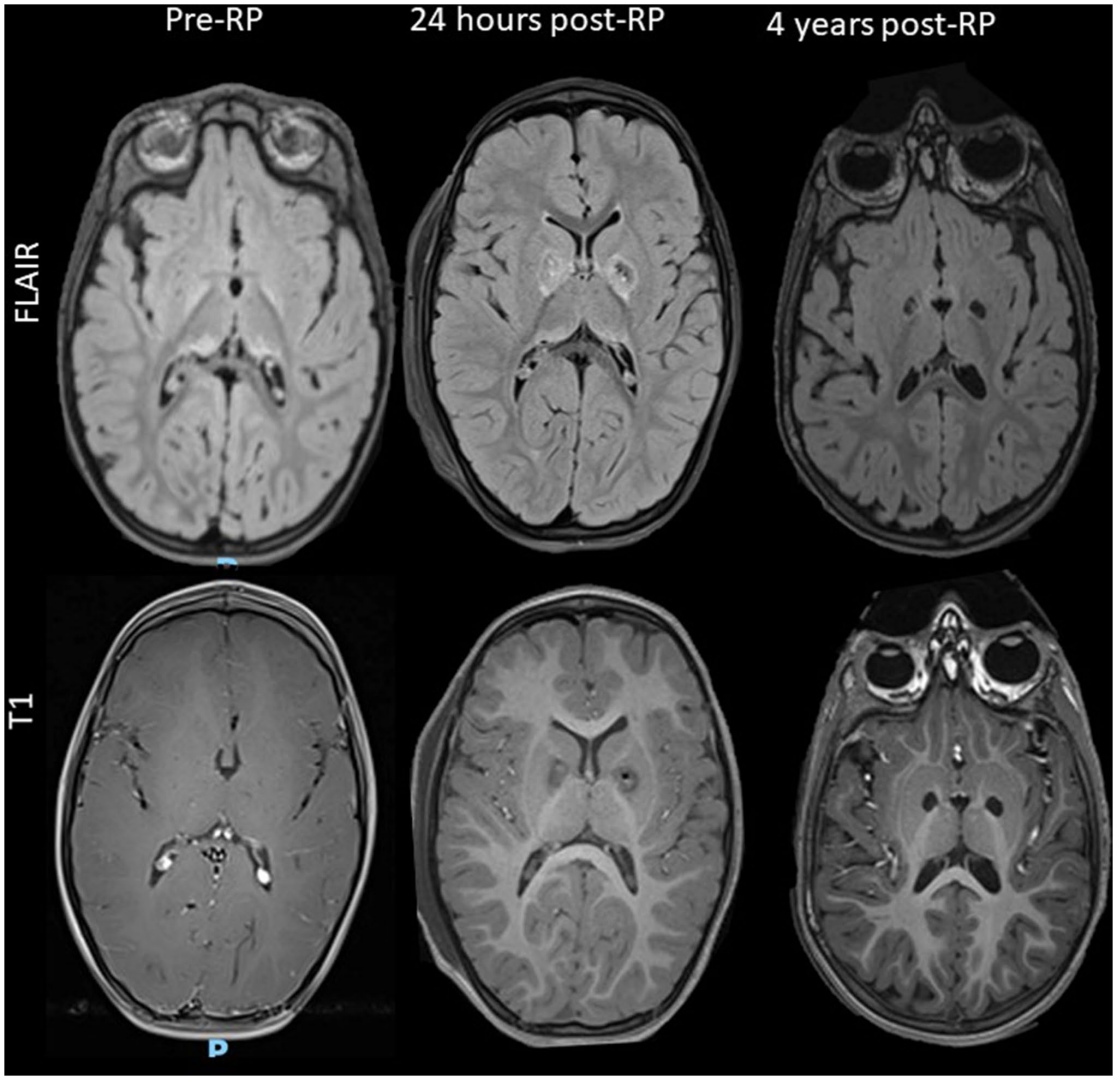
Evolution of MRI imaging after RP in a representative patient (subject #7). Preoperative MRI shows subtle bilateral FLAIR hyperintensities in the globi pallidi and thalami. Early postoperative imaging demonstrates a necrotic core with surrounding diffuse oedema involving the striata and internal capsule, while late MRI depicts cavitating evolution of the lesion with oedema resolution.
